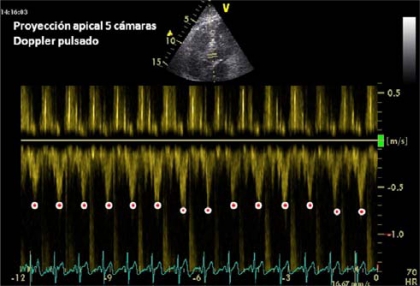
Stroke volume maximum velocity determination (pulsed Doppler in LVOT). This image was collected immediately after a load of 500 cc of 0.9% Saline in a patient with septic shock of biliary tract origin. There was no evidence of significant variability compared to basal imaging, suggesting the need for vasoactive treatment.
